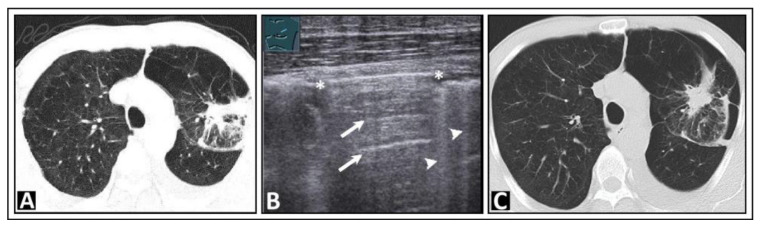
A patient with bronchial carcinoma and localized pneumothorax after lung biopsy. (A) Visualization of a lesion of the left lung on chest computed tomography before biopsy. (B) A localized pneumothorax with two lung puncture points (*), A-lines dorsal to the free air (arrows), and B-lines behind the lung sliding sign (arrowheads). (C) Computed tomography confirms the diagnosis of a pneumothorax.
